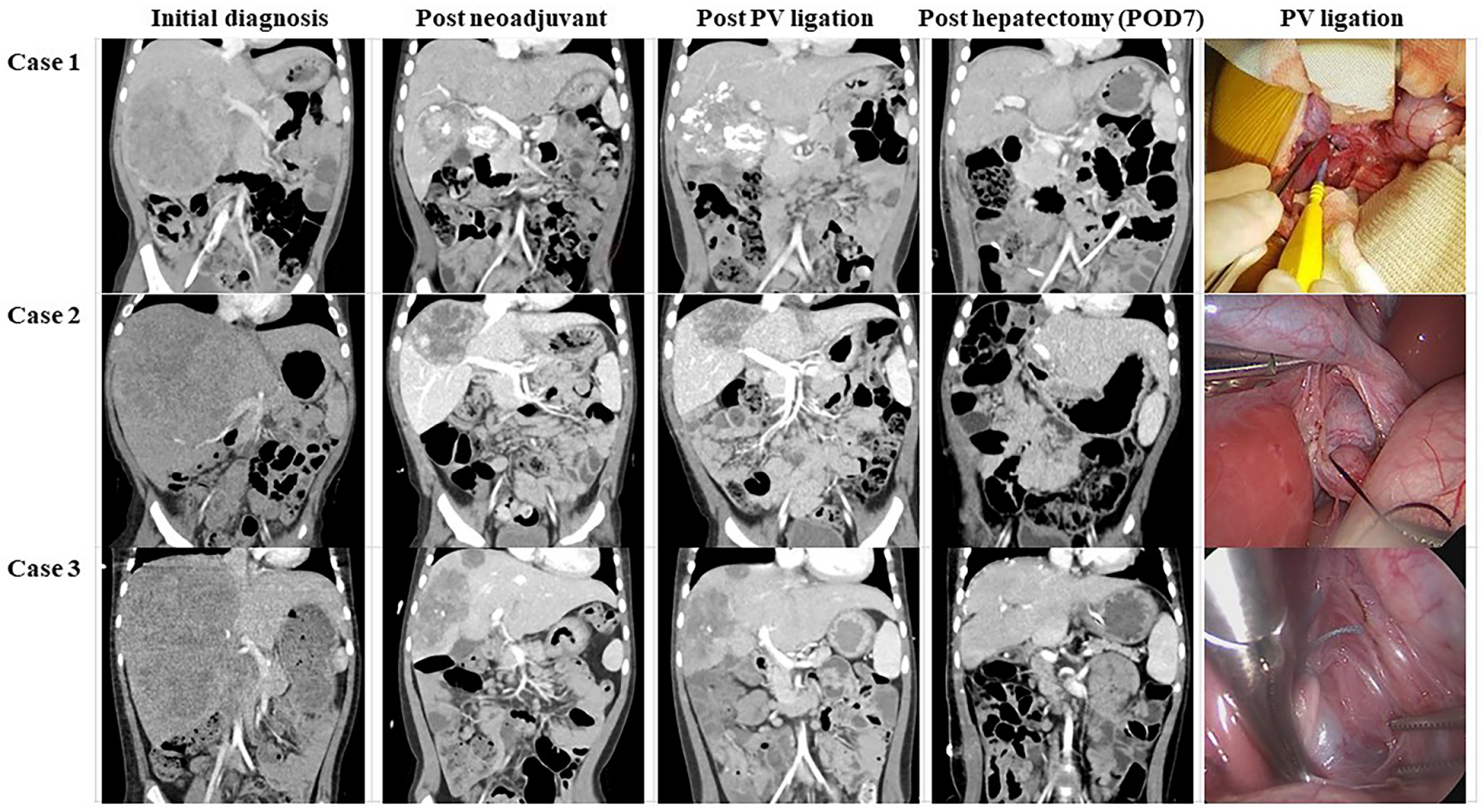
Ct images and intraoperative findings of each patient. The coronal image was used to compare degrees of tumor and liver parenchymal growth. Moreover, intraoperative images revealed that PV ligation was performed without massive dissection or bleeding. *CT, computed tomography; POD, postoperative day(s); PV, portal vein.
